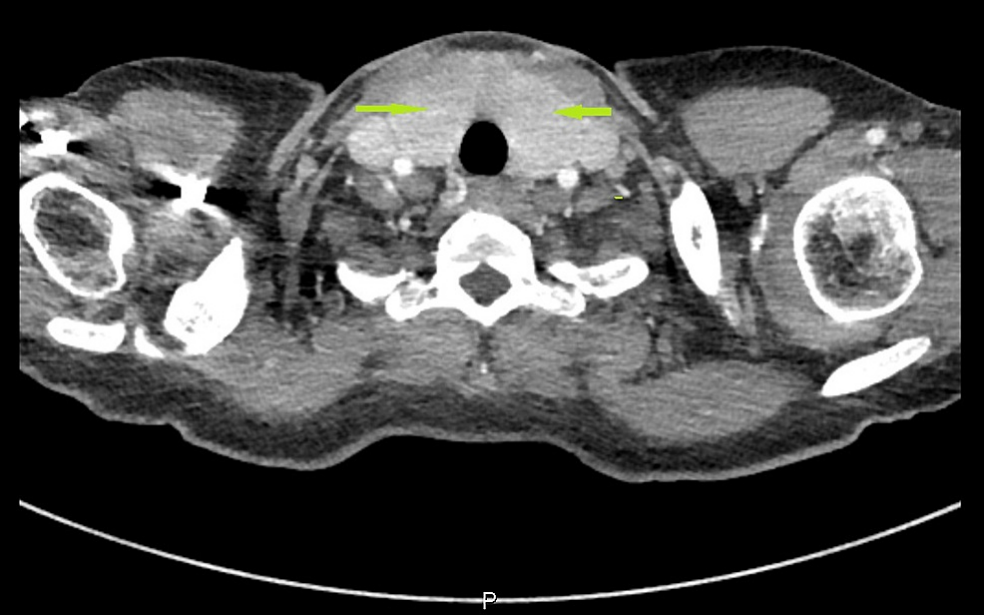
CT imaging showing markedly enlarged heterogeneous thyroid gland with prominent cervical lymphadenopathy (arrows)
